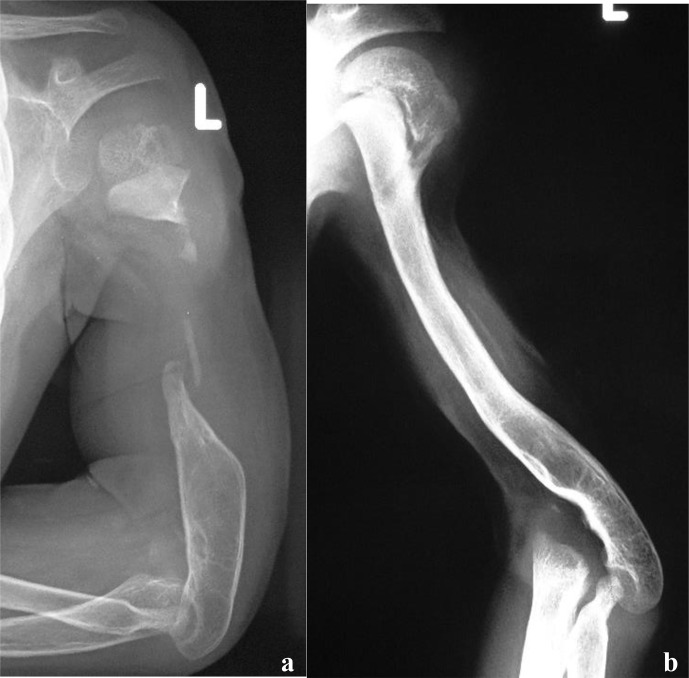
(a) Radiographs, at presentation, of a 2-year-old boy who presented with bone loss and sequelae of septic arthritis of the elbow joint. There was a history of two previous attempts to fill the gap with cortico-cancellous grafts. Reconstruction options were limited by the short stumps, severe osteopenia and the previous clinical history. (b) Radiographs taken at last follow-up, 4 years after VFF; soundly integrated graft with excellent functional results.
